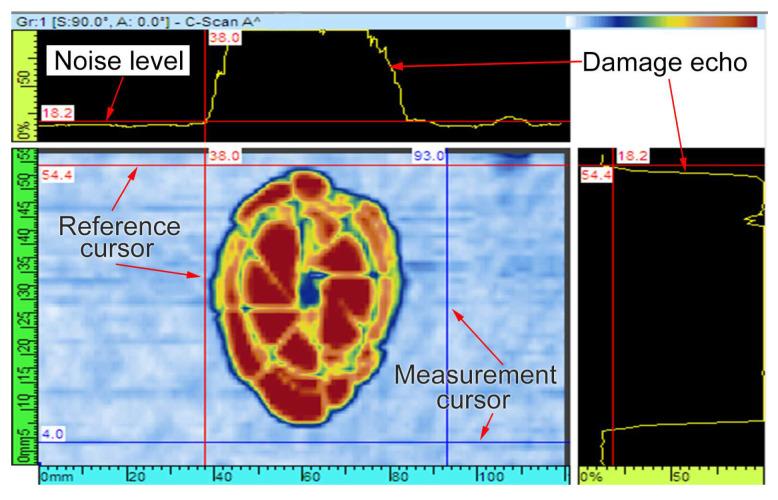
Echo dynamic of the ultrasonic echo.
